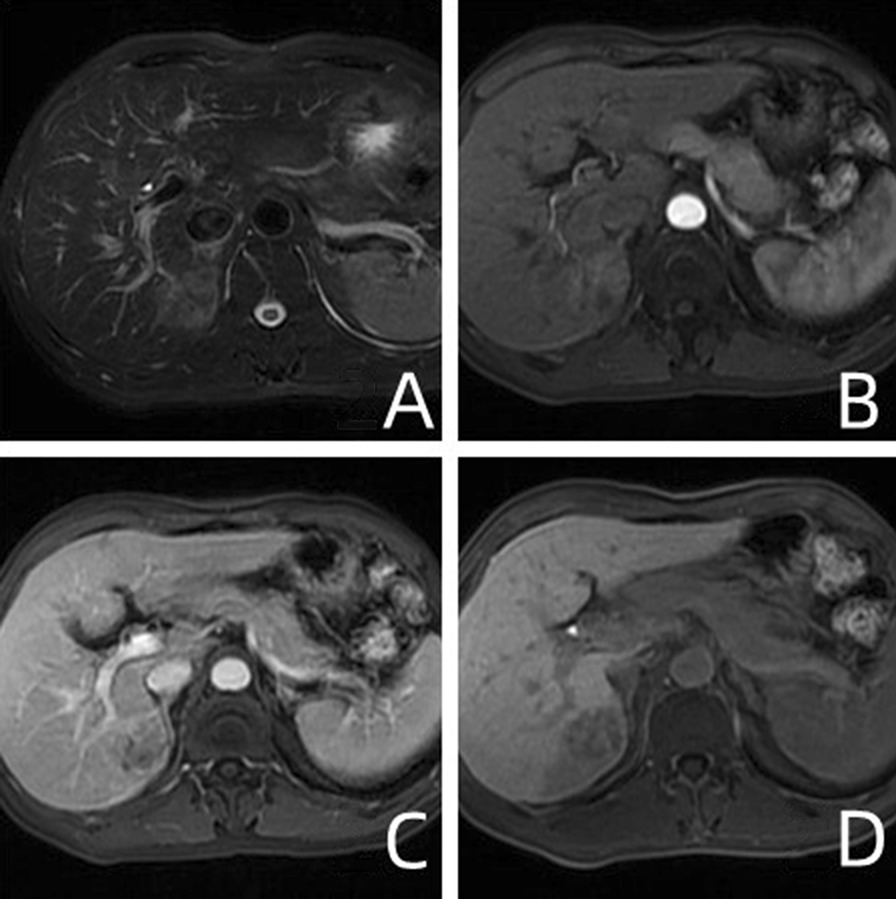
A 43-year-old male with High-grade HCC without MVI. A Unevenly high signals on T2WI; B, C In the arterial phase of GD-Bopta-enhanced MRI, the lesions showed obvious uneven enhancement, and the enhancement was weakened in the delayed phase. D The mass at the hepatobiliary phase has low signal intensity, with a peritumor area of low signal (white arrow)
